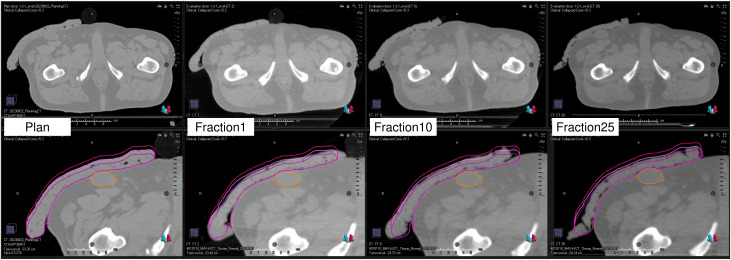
Computed tomography image used for treatment planning and kilovoltage computed tomography images acquired immediately before irradiation at the first, tenth, and twenty-fifth treatment fractions. The lower panels display the bolus contour, ± 5 mm modified contours (external surface only), and the gross tumor volume contour.
